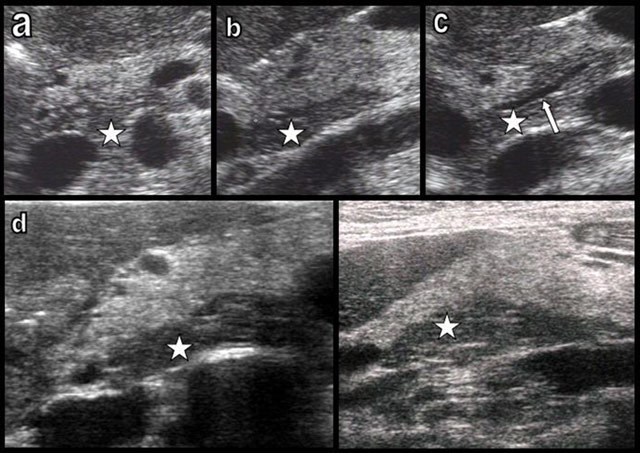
Uneven FI of the cephalic pancreas (type 1a) is commonly found during abdominal ultrasound, especially in middle-aged women. Classical transverse views (a, c, and d) and sagittal views (b and e) are shown. The biliary duct and the distal Wirsung (white arrow) classically run in the PPH (embryological ventral bud), which more commonly appears hypoechoic (star) because it is lesser affected by FI.
